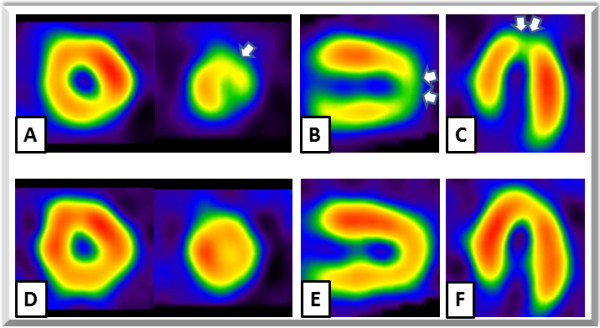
The exercise stress thallium-201 single-photon emission computed tomography. The exercise stress images (A-C) showed moderate defect in apico-anterior wall. The images at rest (D-F) showed redistribution in the same area. They revealed apico-anterior myocardial ischemia.
